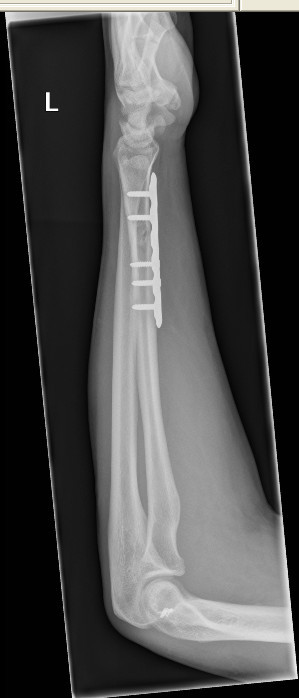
A postoperative X-ray gives a lateral view after open repair of collateral ligaments.
